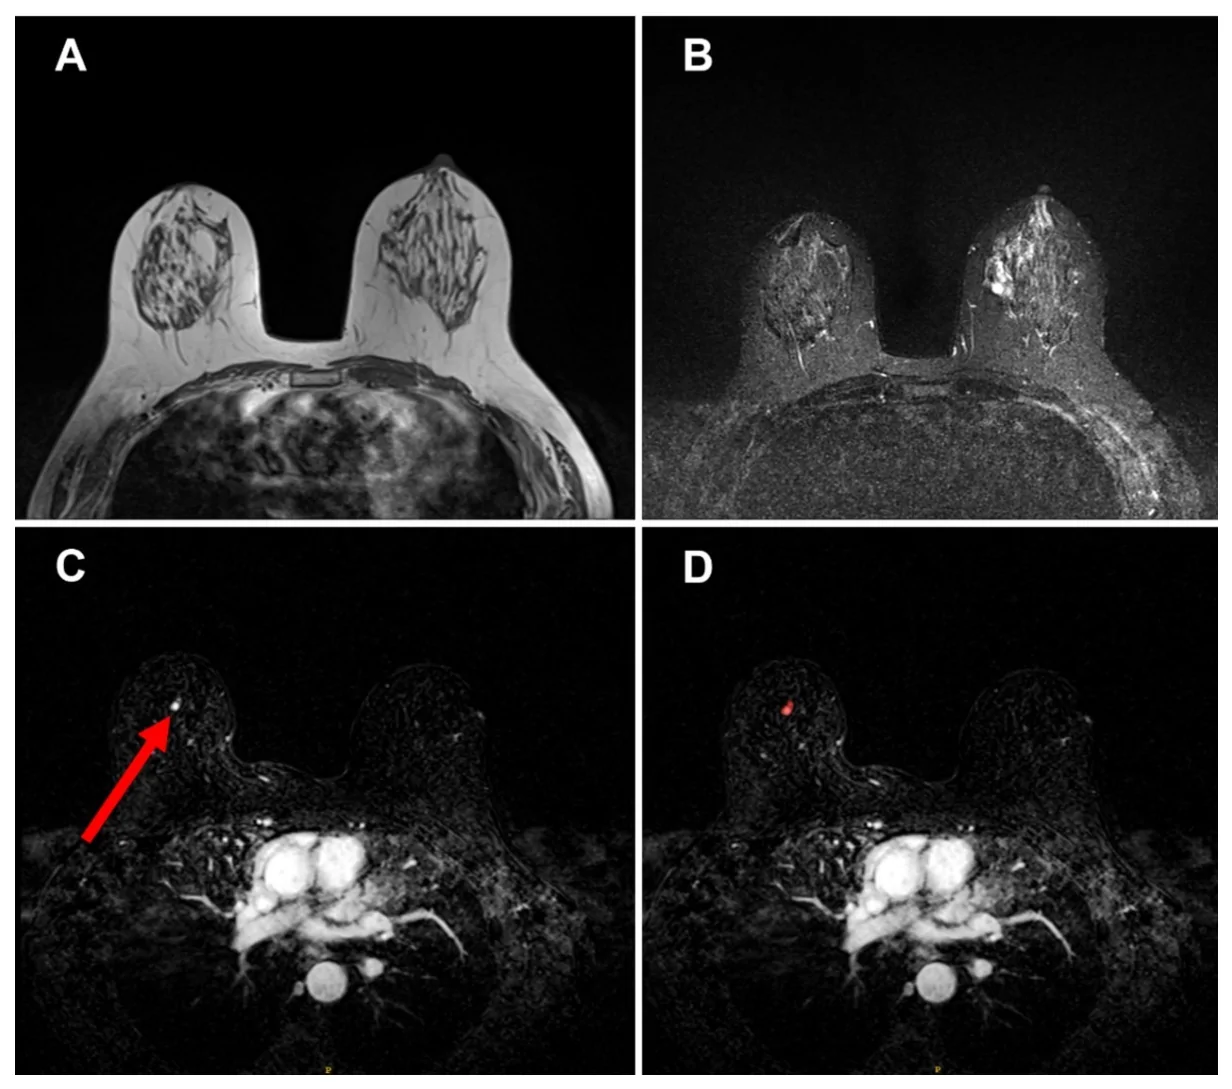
Breast MRI demonstrates focal enhancement. (A) Axial T1-weighted image; (B) axial T2-weighted STIR sequence; (C) axial early-phase post-contrast subtraction image with red arrow indicating the small enhancing lesion; (D) corresponding subtraction image with the enhancing lesion segmented and highlighted in red.
